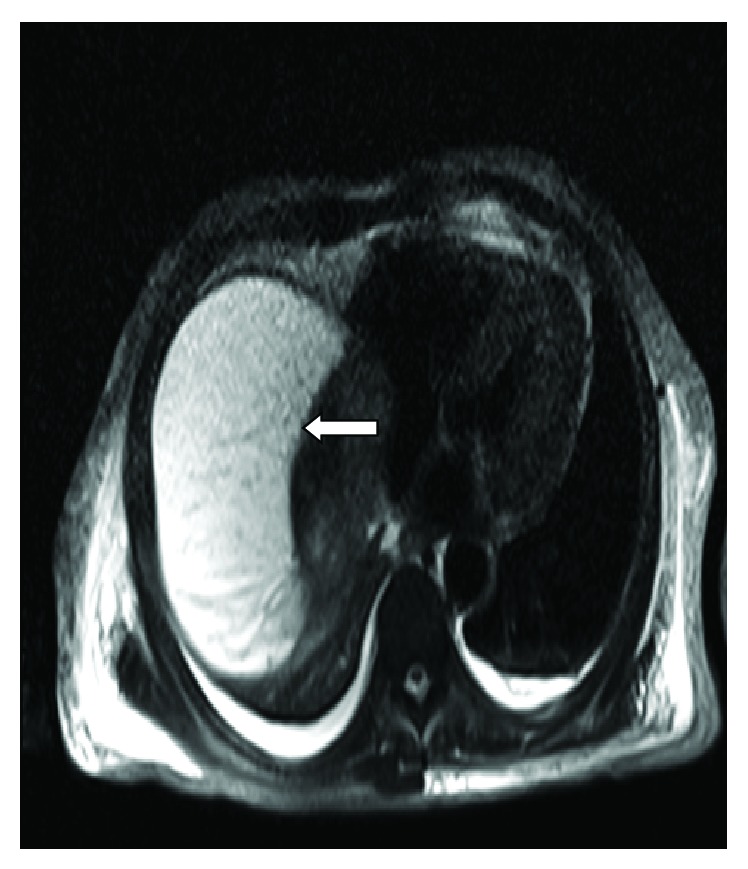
MRI of the abdomen with a large subcapsular abscess.
